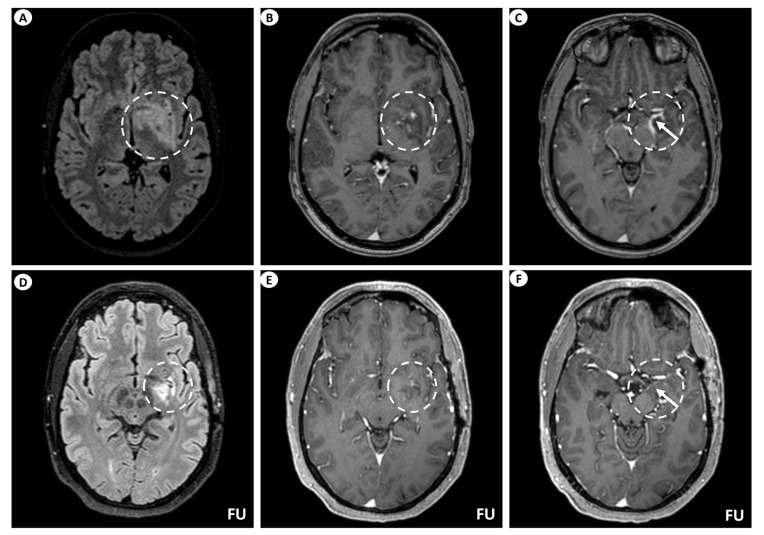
Brain MRI of patient 1. (A) Axial T2-FLAIR (fluid-attenuated inversion recovery) images display signal hyperintensity centered within the basal ganglia (putamen, globus pallidus) and internal capsule on the left (dashed circle). (B) Axial T1-weighted images following intravenous gadolinium injection demonstrate punctate as well as linear contrast enhancement extending along the perivascular spaces (dashed circle), and (C) leptomeningeal contrast enhancement along the surface of the uncus (as indicated by the arrow within the dashed circle). Follow-up (FU) MRI examination in the same patient displays a noteworthy decrease in the extent of the signal hyperintensity within the left basal ganglia on axial T2-FLAIR images (dashed circle) (D). Similarly, axial T1-weighted images following intravenous gadolinium injection demonstrate partial subsidence of the perivascular contrast enhancement (dashed circle) (E), and complete resolution of the leptomeningeal enhancement (arrow within the dashed circle) (F).
